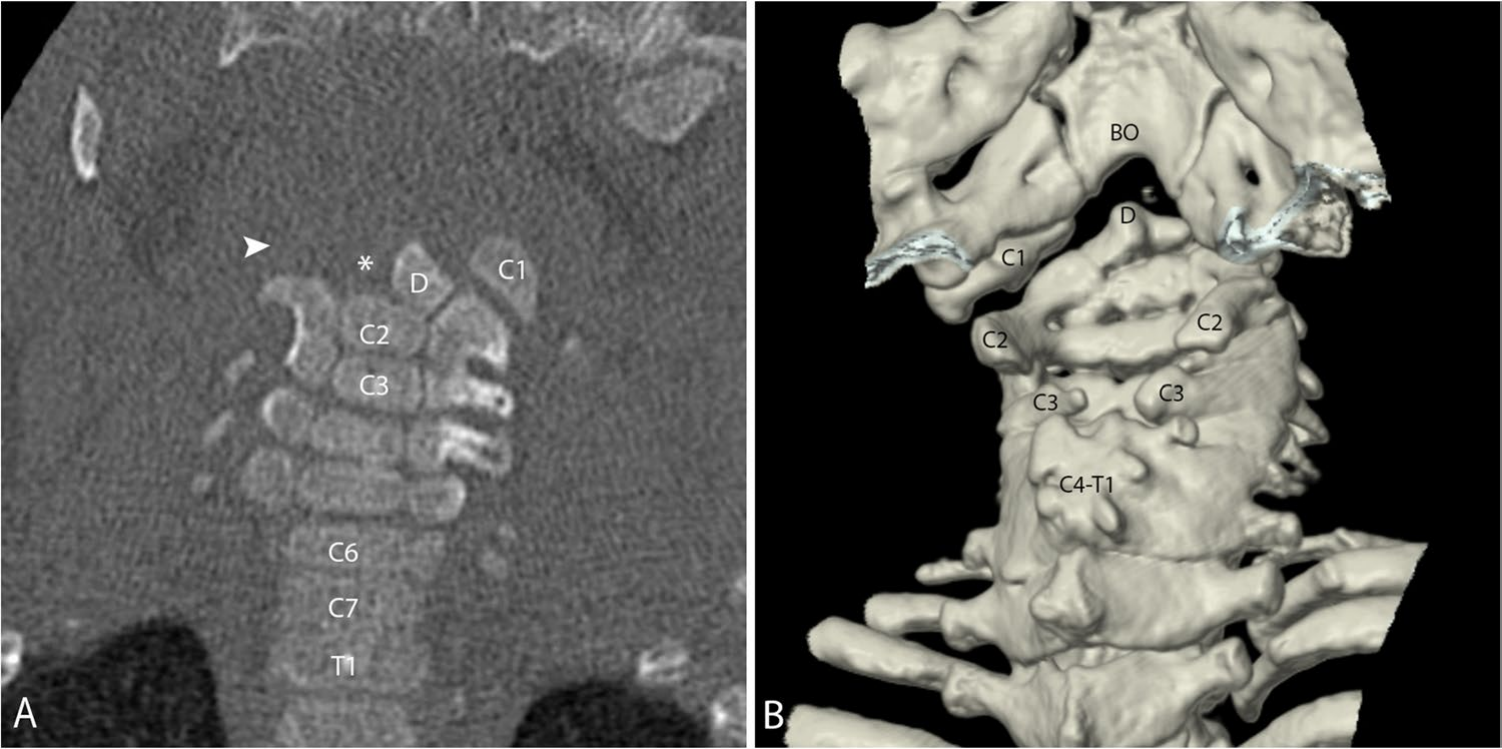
Coronal CT of the brain in a 4-year-old female with Treacher-Collins syndrome. Hypoplasia of the posterior arch of C1 results in a shortened AP dimension of the C1 ring with spinal canal stenosis (*double arrow*). Note the abnormal anterior position of the posterior arch of C1 (*arrowhead*) compared to the posterior arch of C2 (*hollow arrowhead*). As in this case, craniocervical junction findings are often “edge of film” findings on brain imaging
